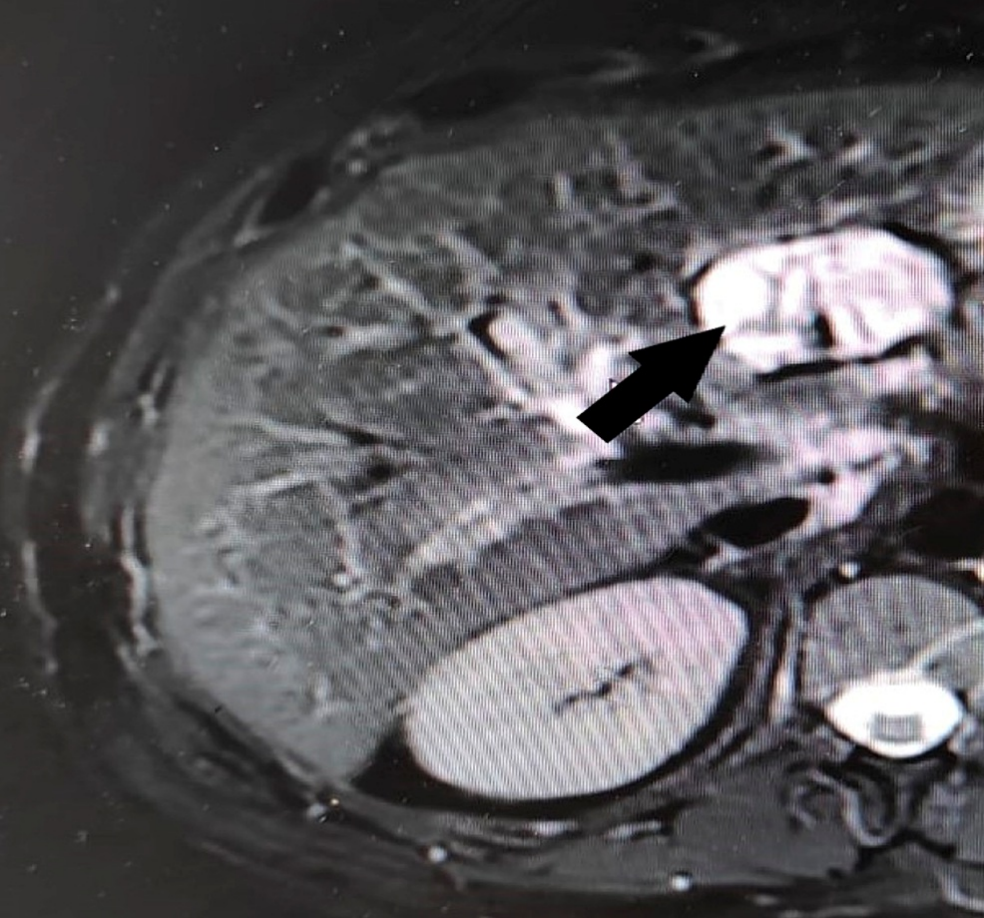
Abdominal MRI showing a serous cyst located in the isthmus of the pancreas
